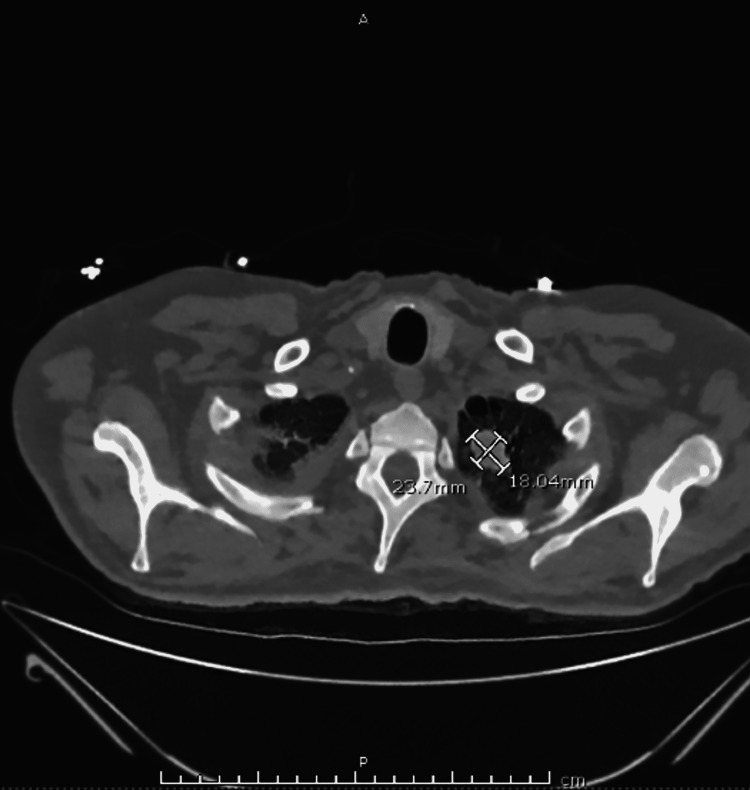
A computed tomography scan showing an increase in the size of a known left upper lobe nodule
